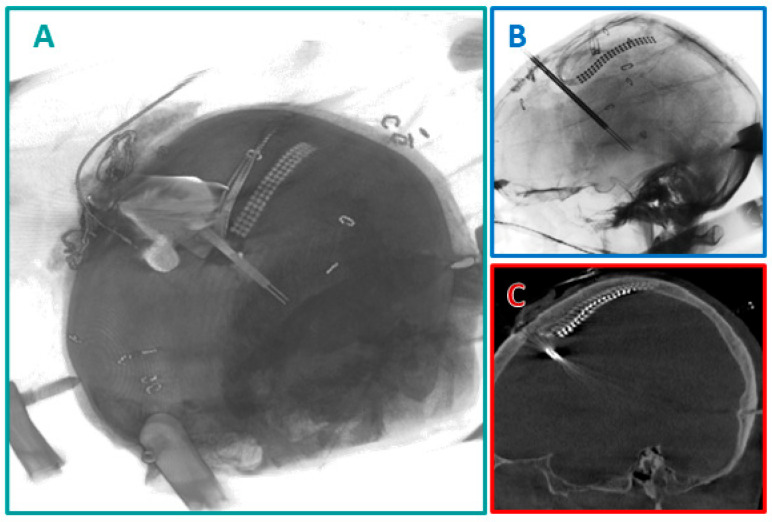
Intraoperative imaging showing the subdural placement of a 63-contact ECoG strip and the DBS leads at their target. (A) 3D reconstruction based on intraoperative CT, (B) Left-sided lateral fluoroscopy image (C), intraoperative CT (O-arm, Medtronic) image.
